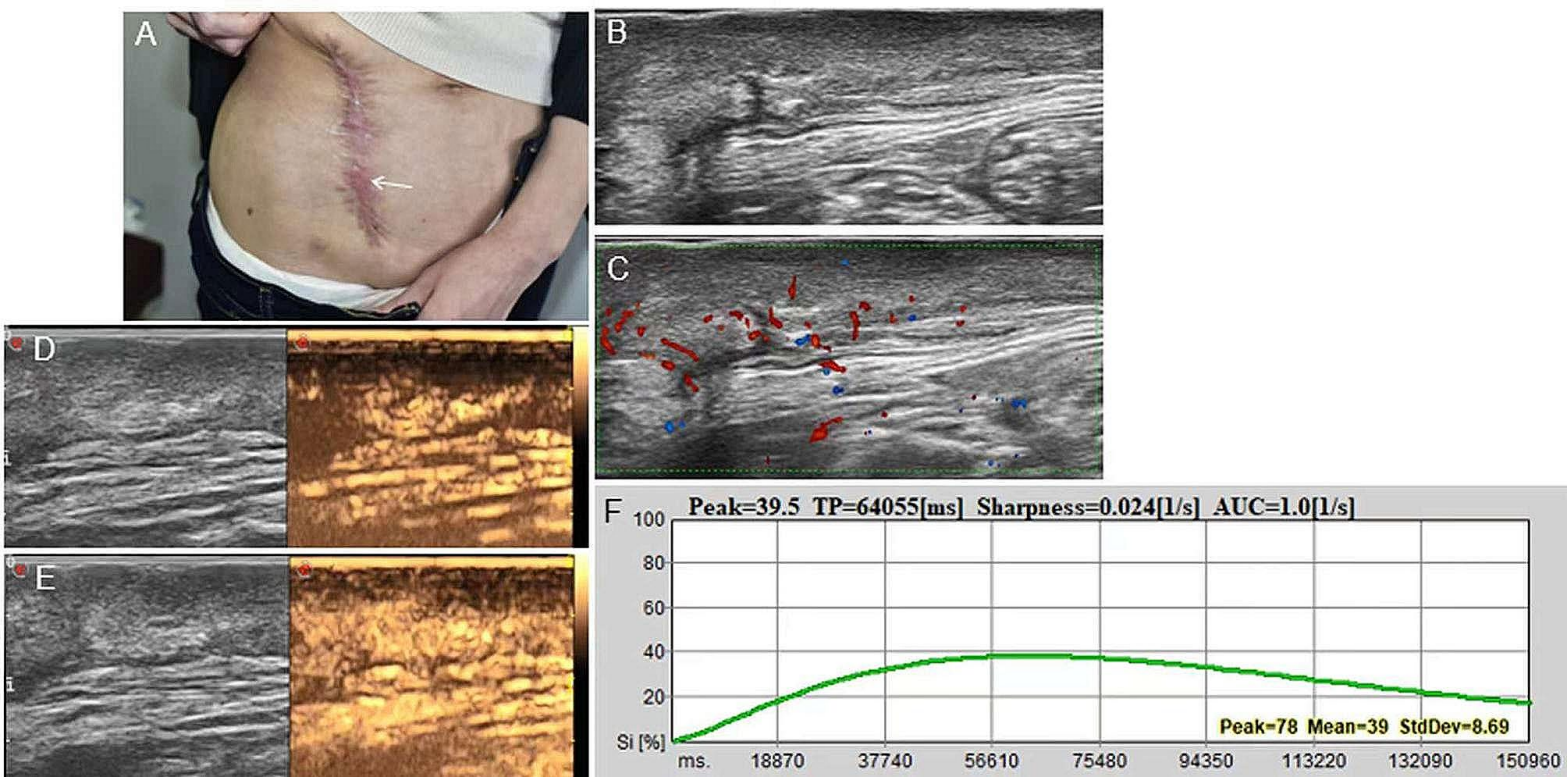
A Post-resection scar with red protrusion in the abdominal wall of a 41-year-old woman. B Transverse ultrasonogram revealing a well-defined, hypoechoic, homogeneous, and subcutaneous lesion. C Color Doppler ultrasonogram revealing rich blood flow signal. D Contrast-enhanced ultrasound revealed the trend that contrast agent enters the lesion from the bottom. E Contrast-enhanced ultrasound revealed homogeneous hyper-enhancement at peak (the 64th second). F Time intensity curve revealed peak of 39.5 and sharpness of 0.024
